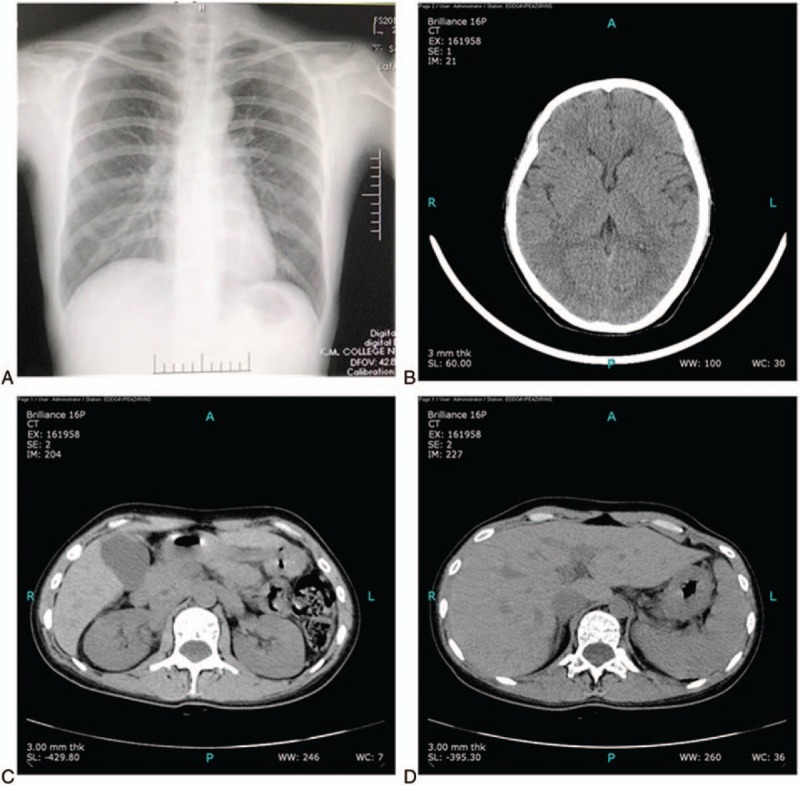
Postoperative CT scan. (A) Chest X-ray was normal. (B) Head CT revealed no abnormalities. (C) Abdominal CT scan of the bilateral kidney was normal. (D) Abdominal CT scan of the left liver lobe showed calcification, and no other abnormalities.
